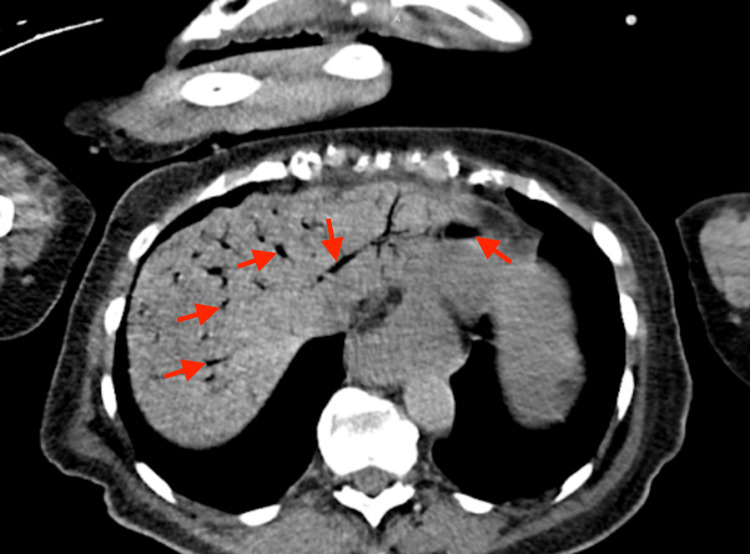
CT Abdomen/Pelvis without contrast (axial view) Diffuse hepatic portal venous gas within the liver (shown by the red arrows).
